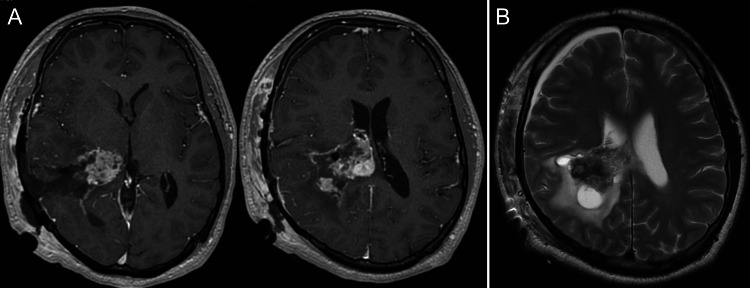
MRI images on postoperative day 5 showing a decrease in tumor enhancement and disappearance of the intratumoral dilated veins Contrast-enhanced T1-weighted axial image on postoperative day 5 showing partial removal of the tumor, and a decrease in enhancement of the residual tumor (A). T2-weighted axial image on postoperative day 5 showing the disappearance of the intratumoral dilated veins that were seen on the initial MRI (B) MRI: magnetic resonance imaging
